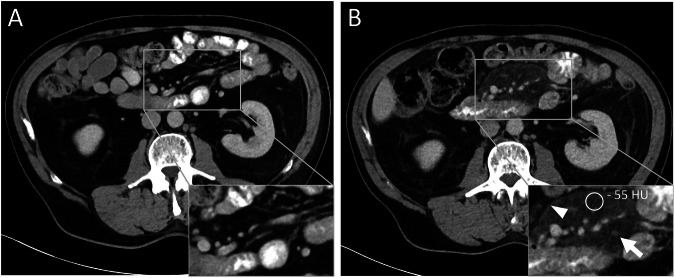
Abdominal CT images of a 72-year-old patient before (A) and 234 days after therapy start with dabrafenib/trametinib, showing newly developed mesenteric panniculitis (B). Arrowhead indicates pseudo-capsule, arrow points at mesenteric nodules and circular ROI shows increased density of the mesenteric fat. HU, Hounsfield units
